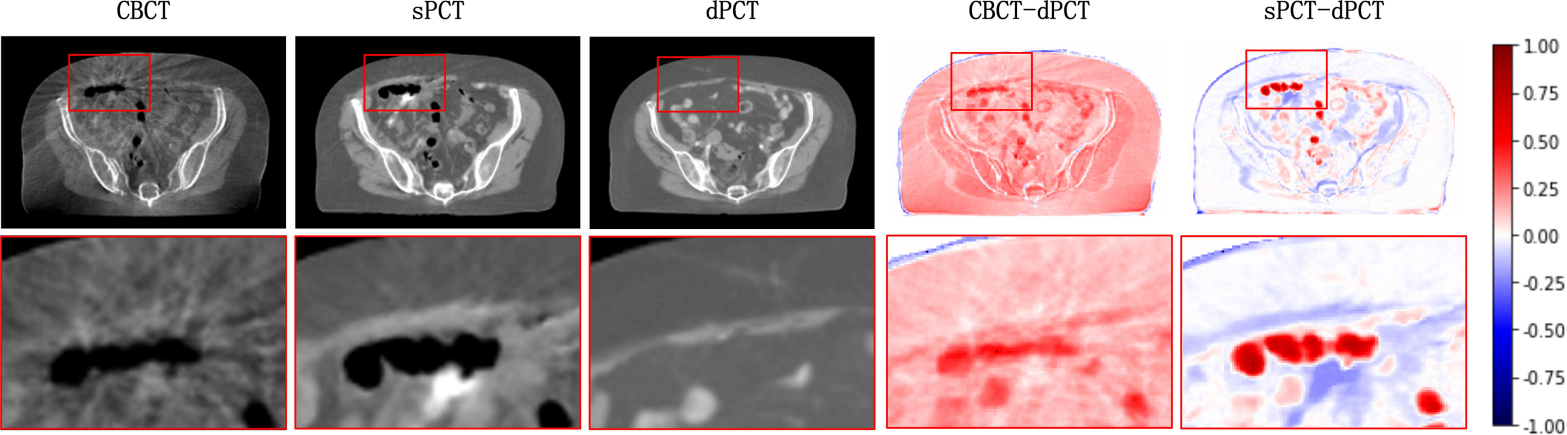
Artifact correction comparison. From left to right are CBCT, sPCT, dPCT, CBCT- dPCT, and sPCT - dPCT. The second row shows an enlarged version of the red rectangle of the image on the first row.
